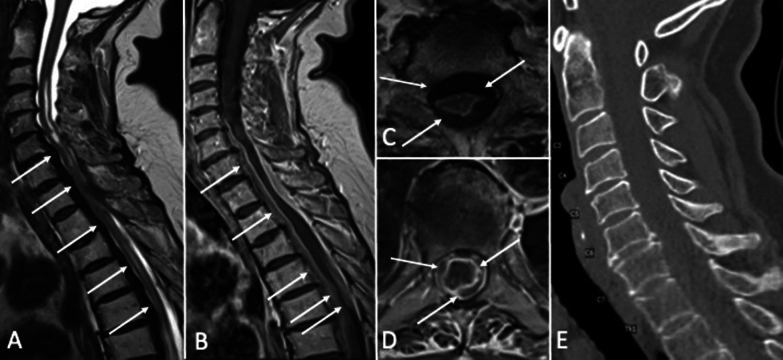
Myelopathy secondary to marked dural thickening. A, C T2-weighted images showing hypointense circular dural thickening extending over 13 vertebral segments (arrows) causing compressive myelopathy. B, D Contrast enhanced T1-weighted images showing enhancement of the dural abnormality (arrows). E Sagittal CT reconstruction shows no bony abnormality within the spinal canal
